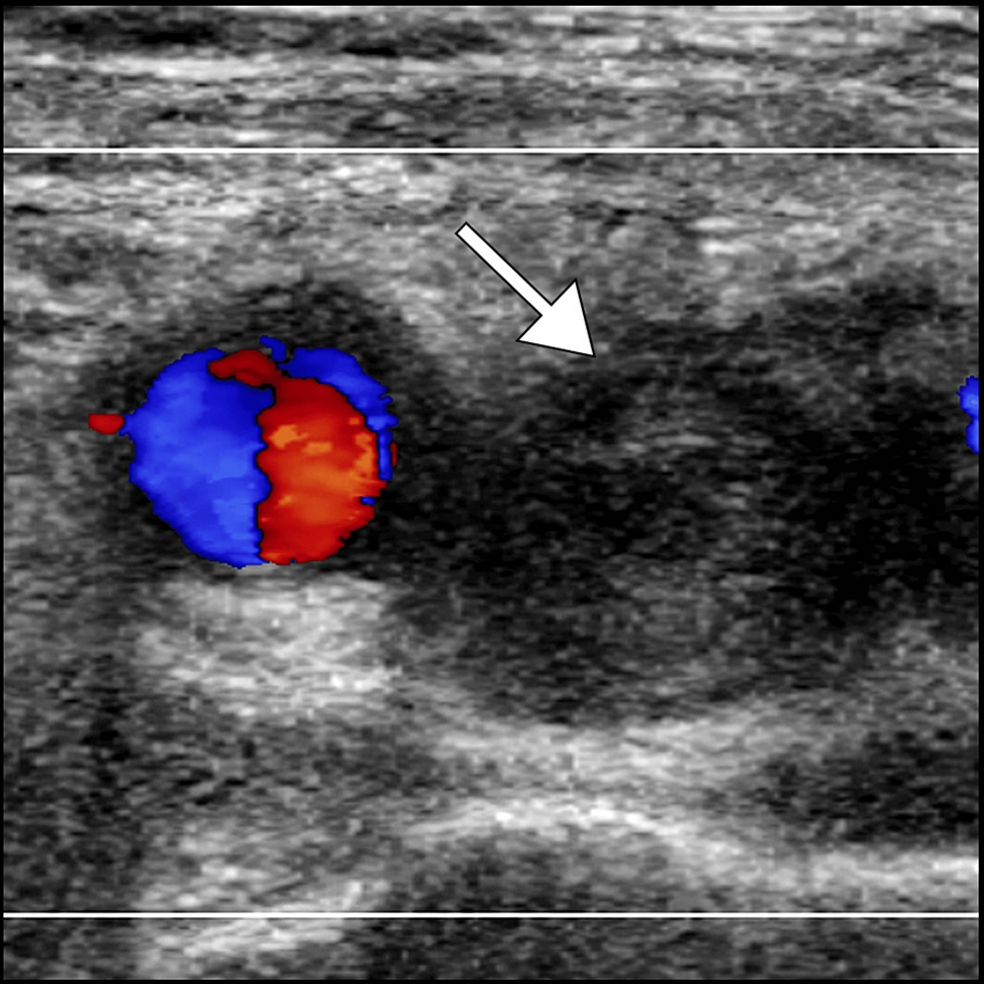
Color Doppler ultrasound image depicting a distended left common femoral vein (arrow) with absent flow, confirming the presence of deep vein thrombosis.
